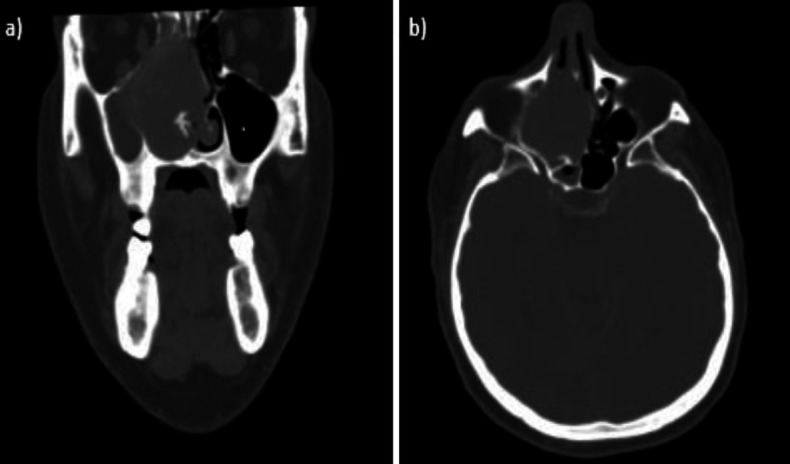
a) CT image of the SFT in coronal section, b) CT image of the SFT in axial section
